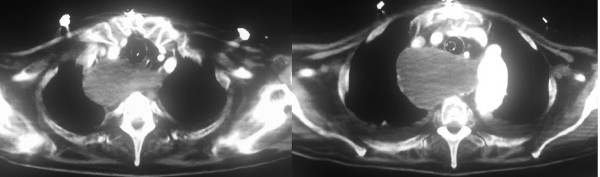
Post-operative CT scan demonstrating successful surgical reconstruction.
